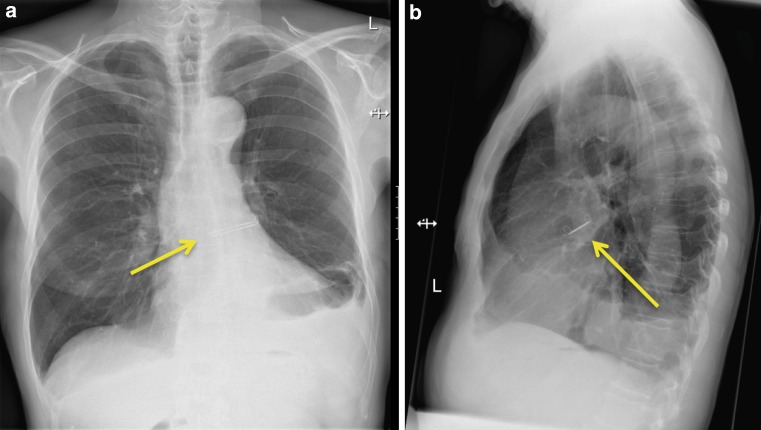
X-ray displaying the position of the Atriclip PRO device
